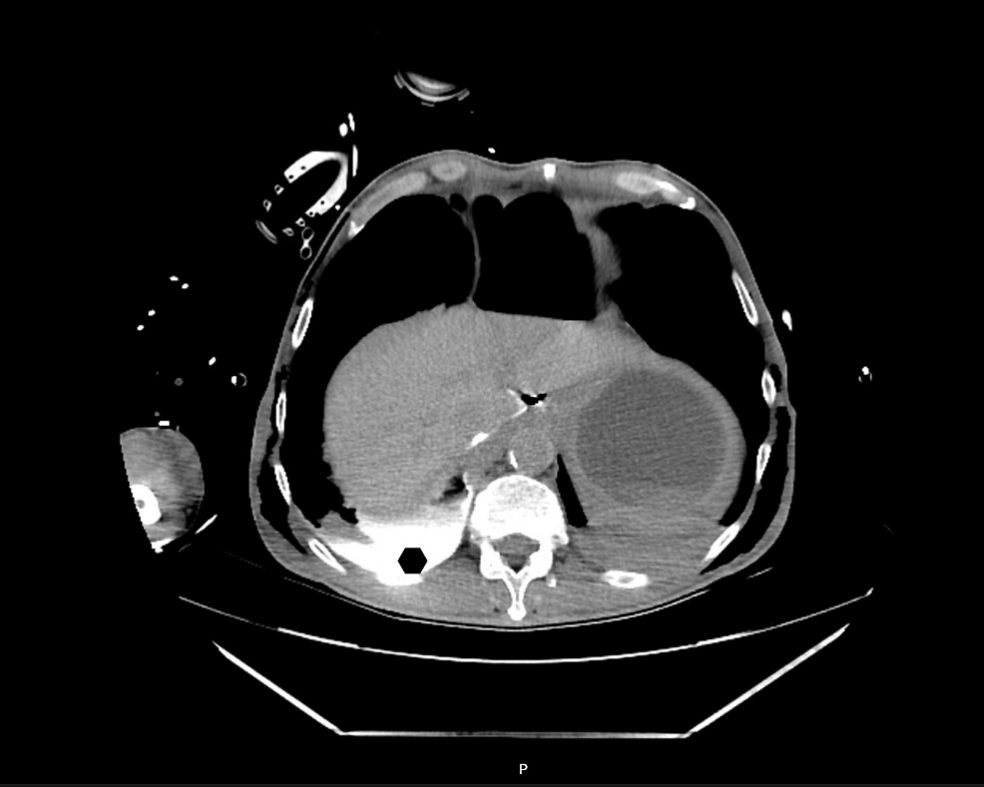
Second CT scan after the thoracic drainage technique Contrast marker on the pleural cavity clearly seen on the posterior right hemithorax (lozenge).
